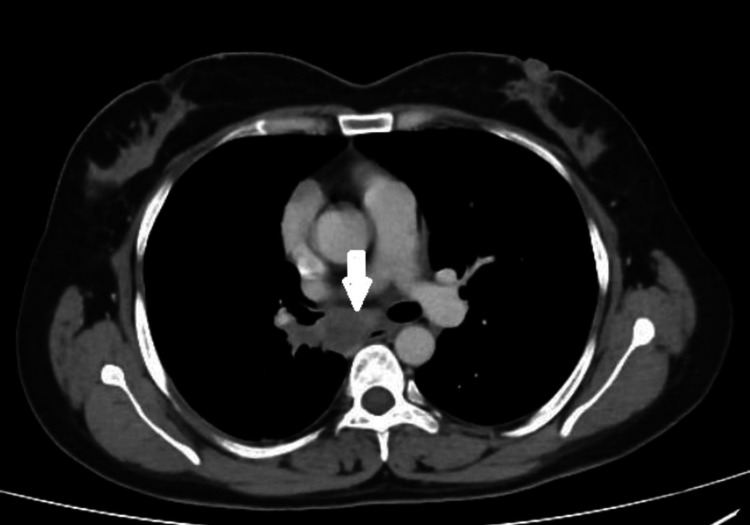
A section of the contrast enhanced CT Chest of a patient diagnosed with breast carcinoma, showing enlarged subcarinal or station 7 lymph node (white arrow)
